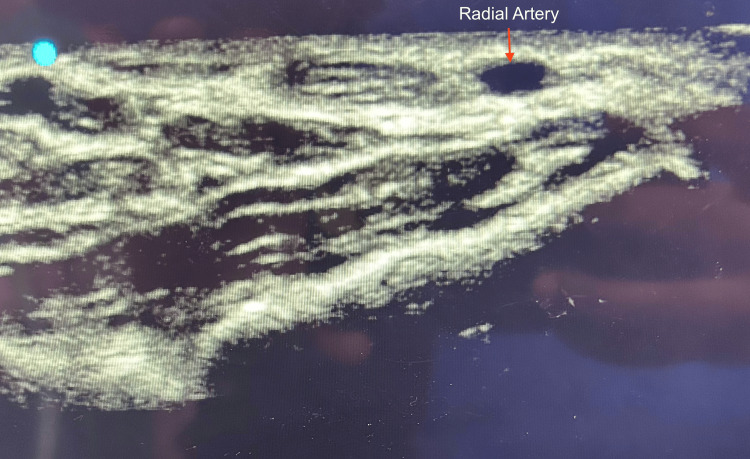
Ultrasound view of radial artery (transverse section)
